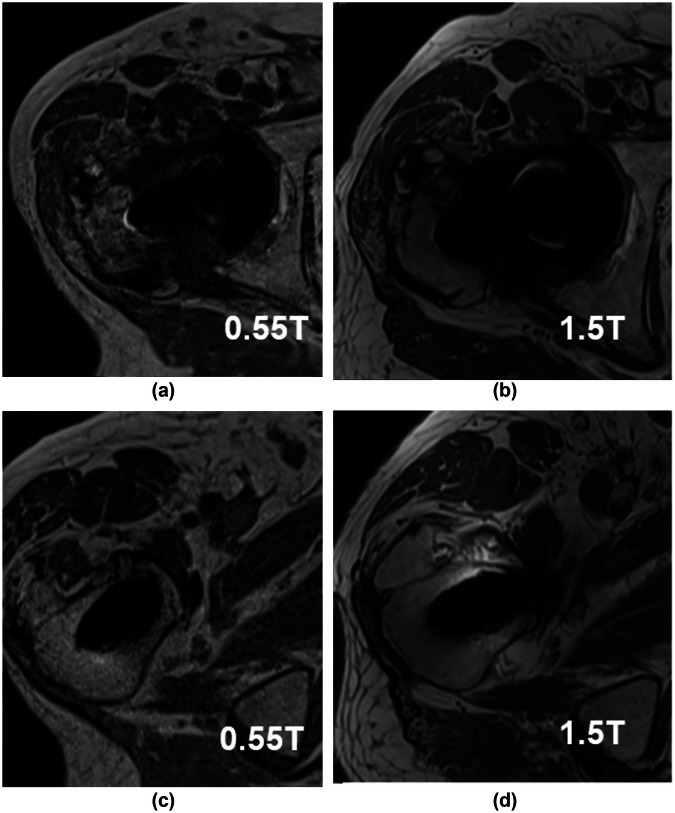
Comparison of artifacts at the level of the acetabulum (a, b) and proximal stem (c, d) at 0.55 and 1.5-T in the same patient on T1-weighted imaging. Reprinted with permission from Breit et al and Elsevier under the Creative Commons CC-BY license [53]
